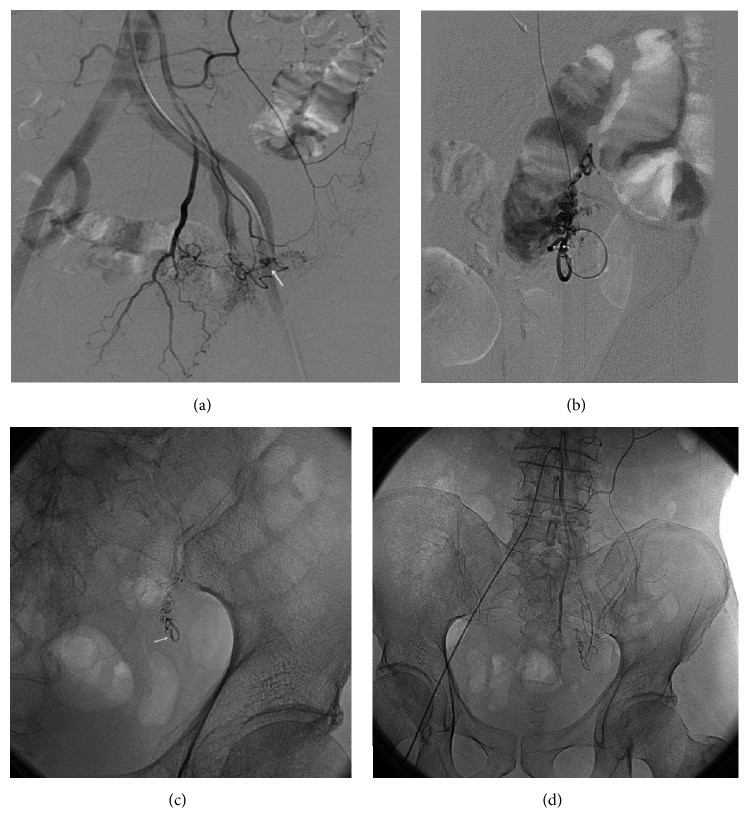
The radiography in the patient (Case 7) with lower gastrointestinal bleeding and angiodysplasia of sigmoid colon. (a) The preoperative radiography shows vascular malformation of sigmoid colon (as showed by the arrow). (b) The superselective radiography was used to evaluate the dose and velocity of the embolization agents. (c) It shows the deformed vascular mass (as showed by the arrow). (d) The postoperative radiography (without digital subtraction) showed that the abnormal sign disappeared.
